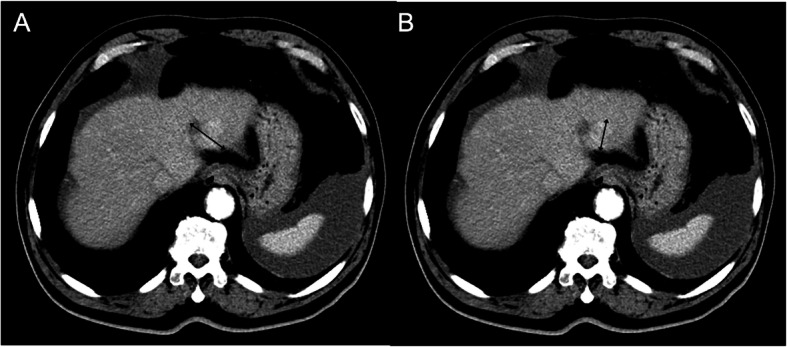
Application of modified Response Evaluation Criteria in Solid Tumors (mRECIST) for hepatocellular carcinoma (HCC). A Measurement of the longest overall target tumor diameter (41 mm) according to conventional RECIST. B Measurement of the longest viable tumor diameter (30 mm) based on tumor enhancement area on arterial-phase CT imaging according to mRECIST for HCC
